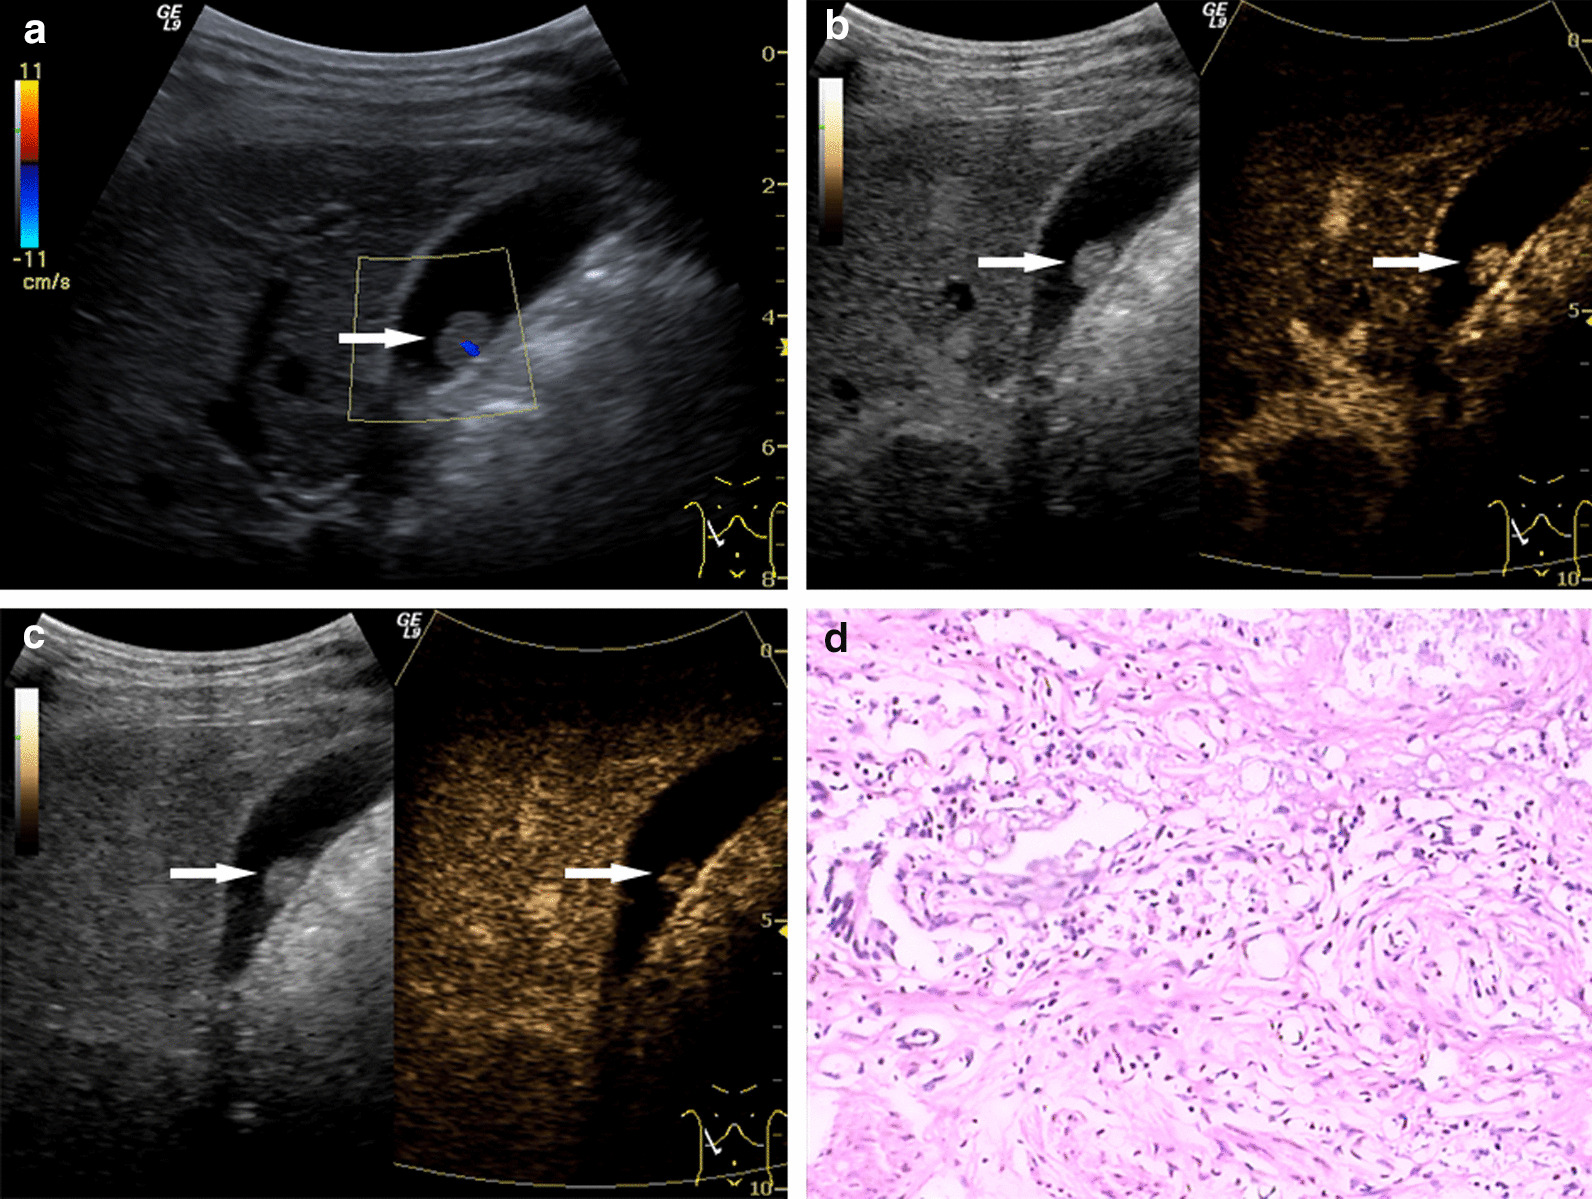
Gallbladder adenoma. a Ultrasonography (US) showed an isoechoic lesion, 1.0 × 0.7 cm in size, with detectable blood flow on Color Doppler flow imaging. b, c On contrast-enhanced ultrasound (CEUS), the lesion showed synchronous enhancement with the gallbladder (GB) wall at 18 s in the arterial phase (b) and gradually showed hypo-enhancement at 38 s, slightly in advance of the GB wall (c). d Postoperative pathological results: GB adenoma
